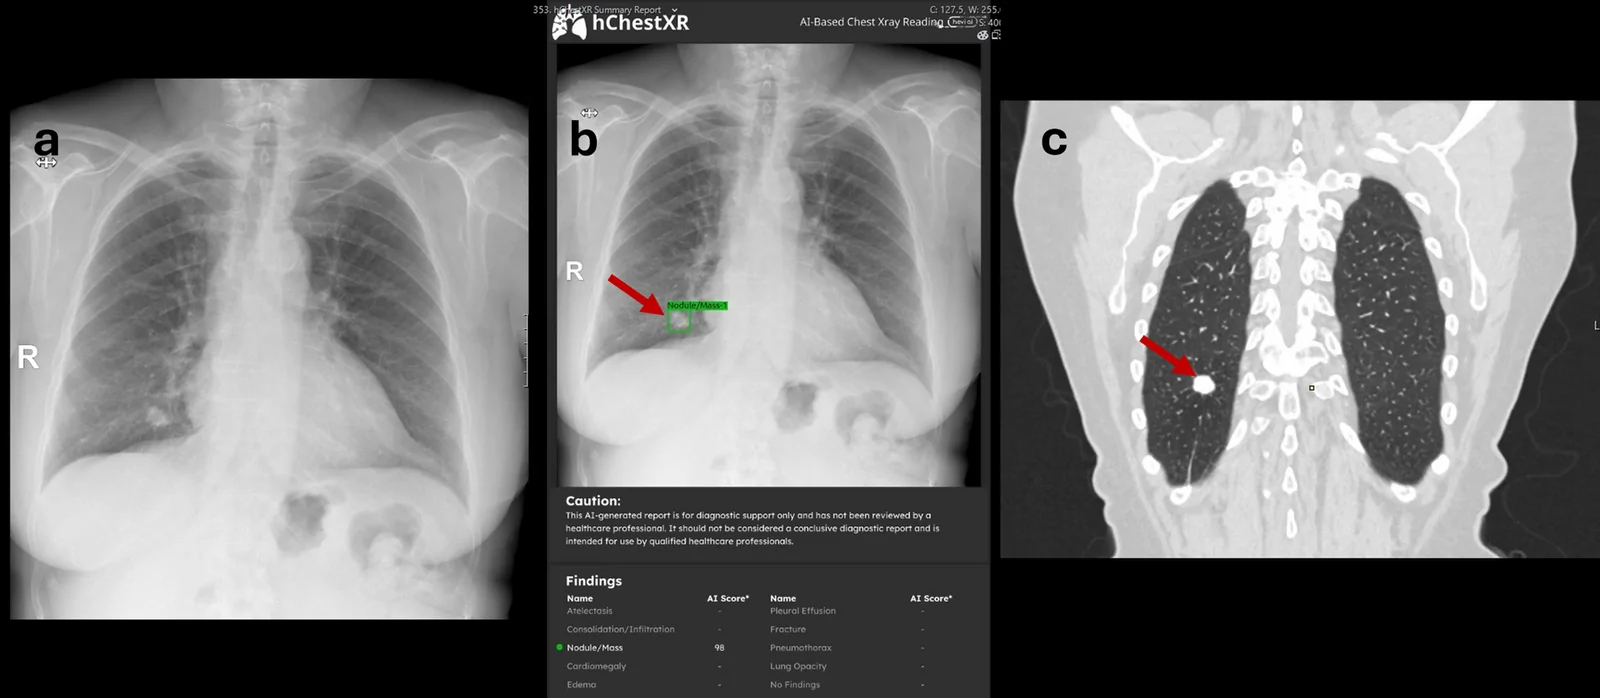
A 69-year-old female patient underwent a chest x-ray exam for a routine check-up in center A. (a) Posteroanterior chest radiograph of the patient. (b) The AI system identified a nodule/mass in the right lower lung zone (arrow). (c) The corresponding coronal CT image demonstrates a nodule in the right lower lobe (arrow). This case was classified as a true positive for the AI system. The subsequent biopsy confirmed lung cancer.
